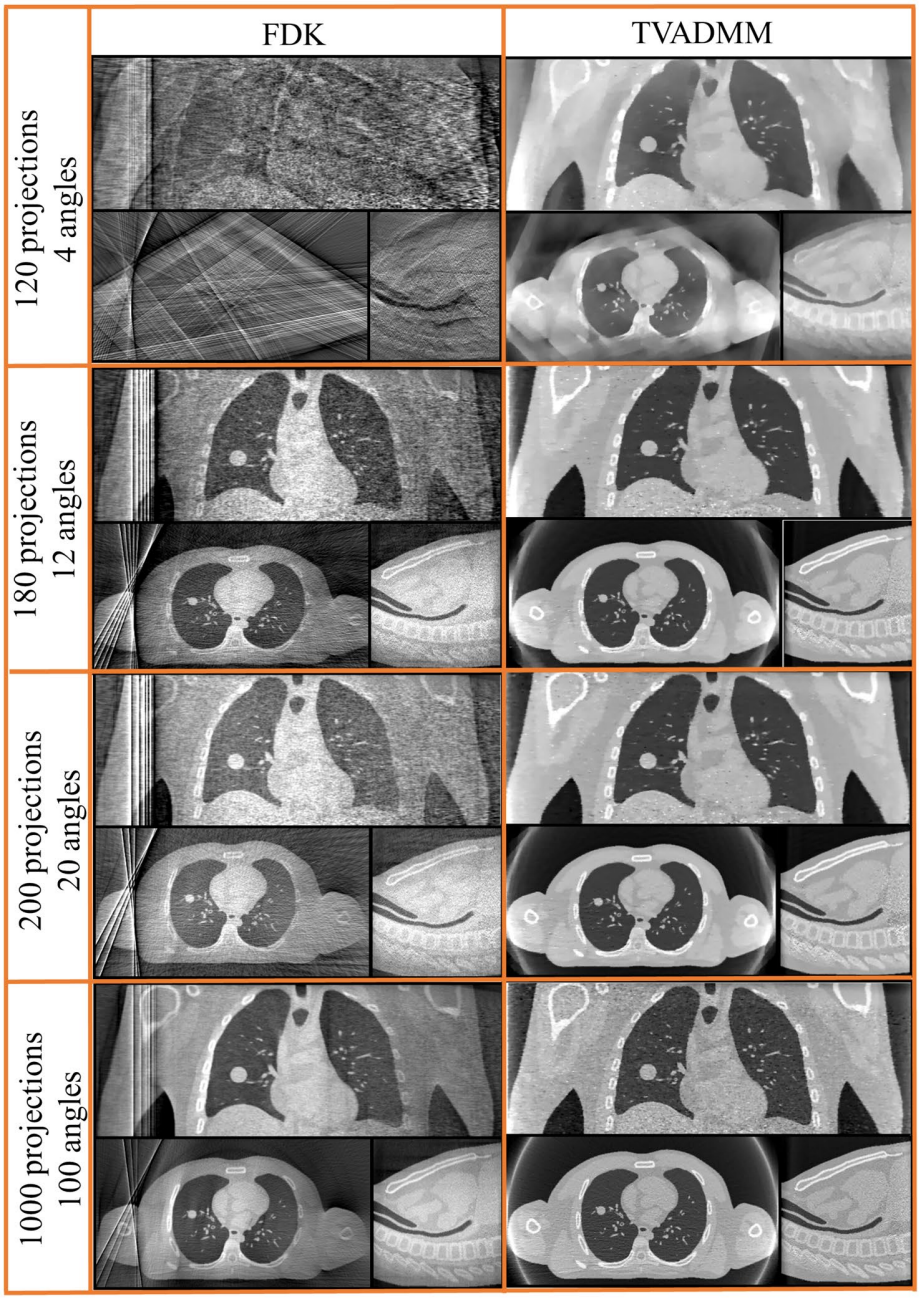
Reconstructed pre-treatment source array DIBH CBCT images for a variety of scan configurations. Note that e.g. 120 projection 4 angular position refers to moving the arrays to 4 distinct angular positions, then acquiring 30 projections from 30 sources along each array at each angular position for 120 projections acquired in total.
